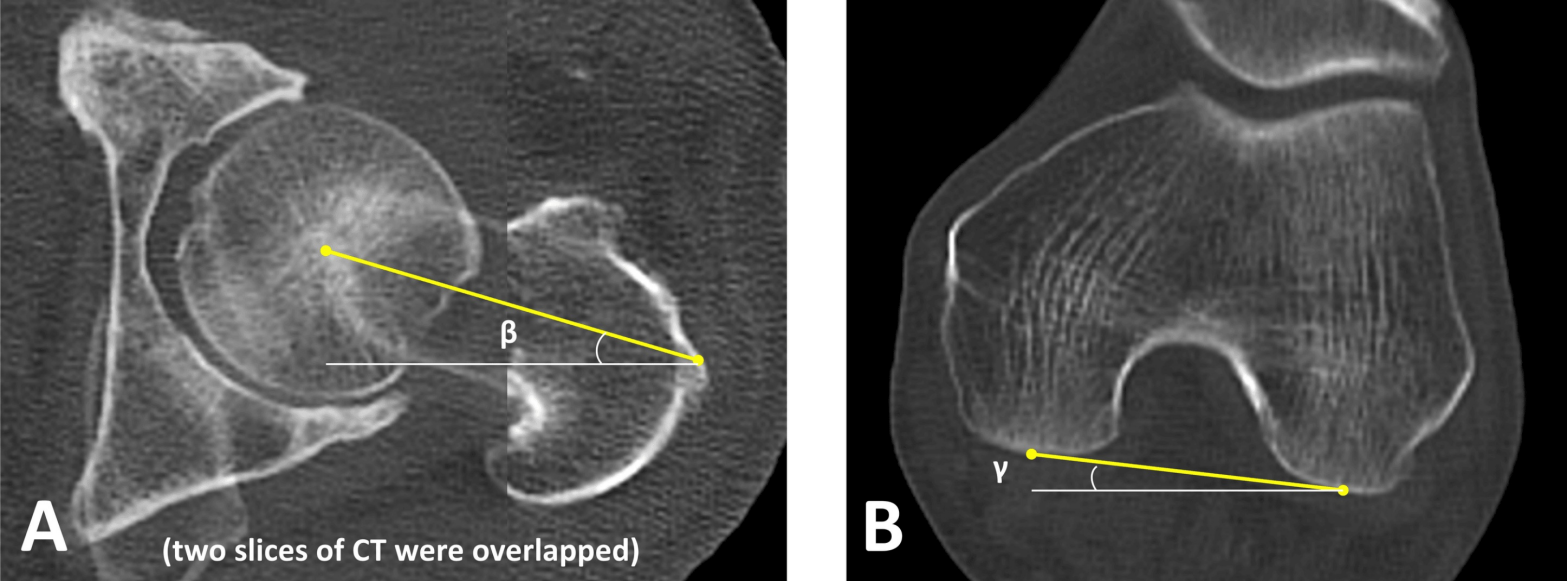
Computed tomography anteversion Computed tomography anteversion (CTAV) was measured as the angle formed between the line intersecting the femoral neck axis and the posterior condylar line of the distal femur. The femoral neck axis was defined as the line connecting the center of the femoral head to the neck bisector on two CT slices where the widest parts of the femoral head and neck were evident (A). The posterior condylar line of the distal femur is defined as the line connecting both edges of the posterior condyles (B). CTAV was calculated as “β minus γ ” in this case.
